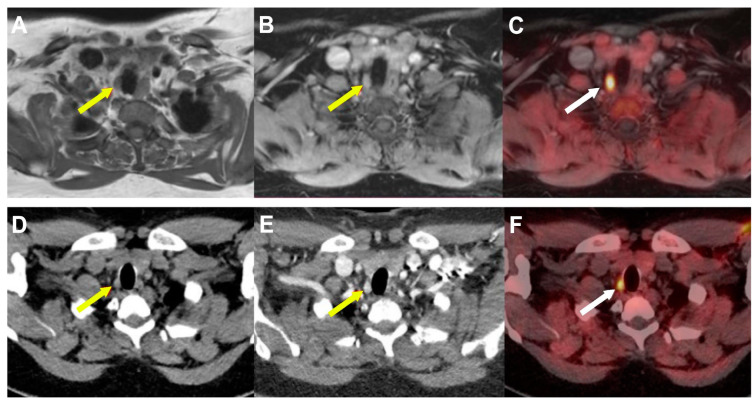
Concordant results of [^18^F]FCH PET/4D-MRI and [^18^F]FCH PET/4D-CT (axial slices) in patient with primary hyperparathyroidism showing right inferior parathyroid tumor (arrows) with high [^18^F]FCH uptake, and early and intense contrast media enhancement ((A): T2-weighted MRI; (B): arterial phase T1-weighted MRI; (C): PET/MRI fusion images; (D): no contrast media enhanced CT; (E): contrast media enhanced CT (arterial phase); (F): PET/CT fusion images). Parathyroid adenoma confirmed after surgery.
